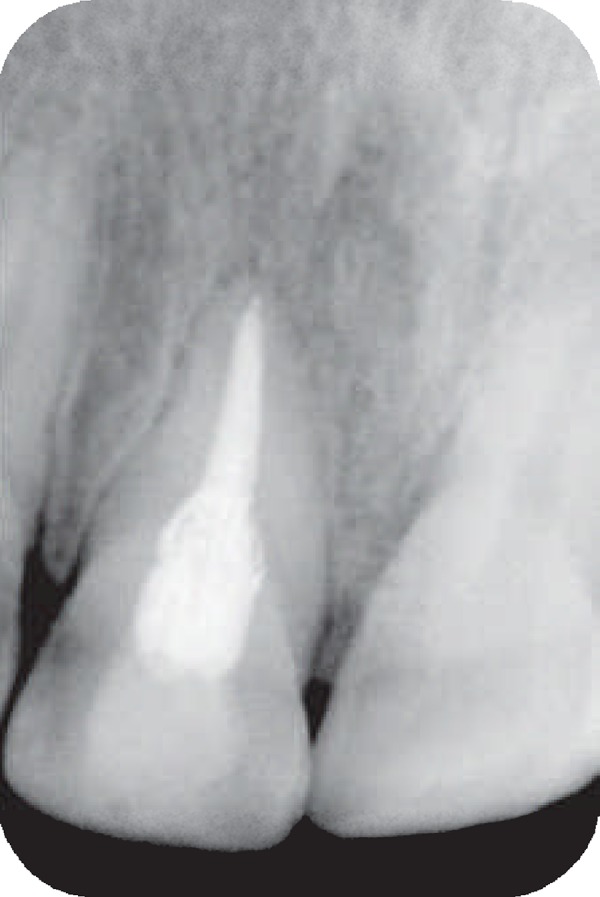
Healing of periapical lesion one year after root canal therapy
